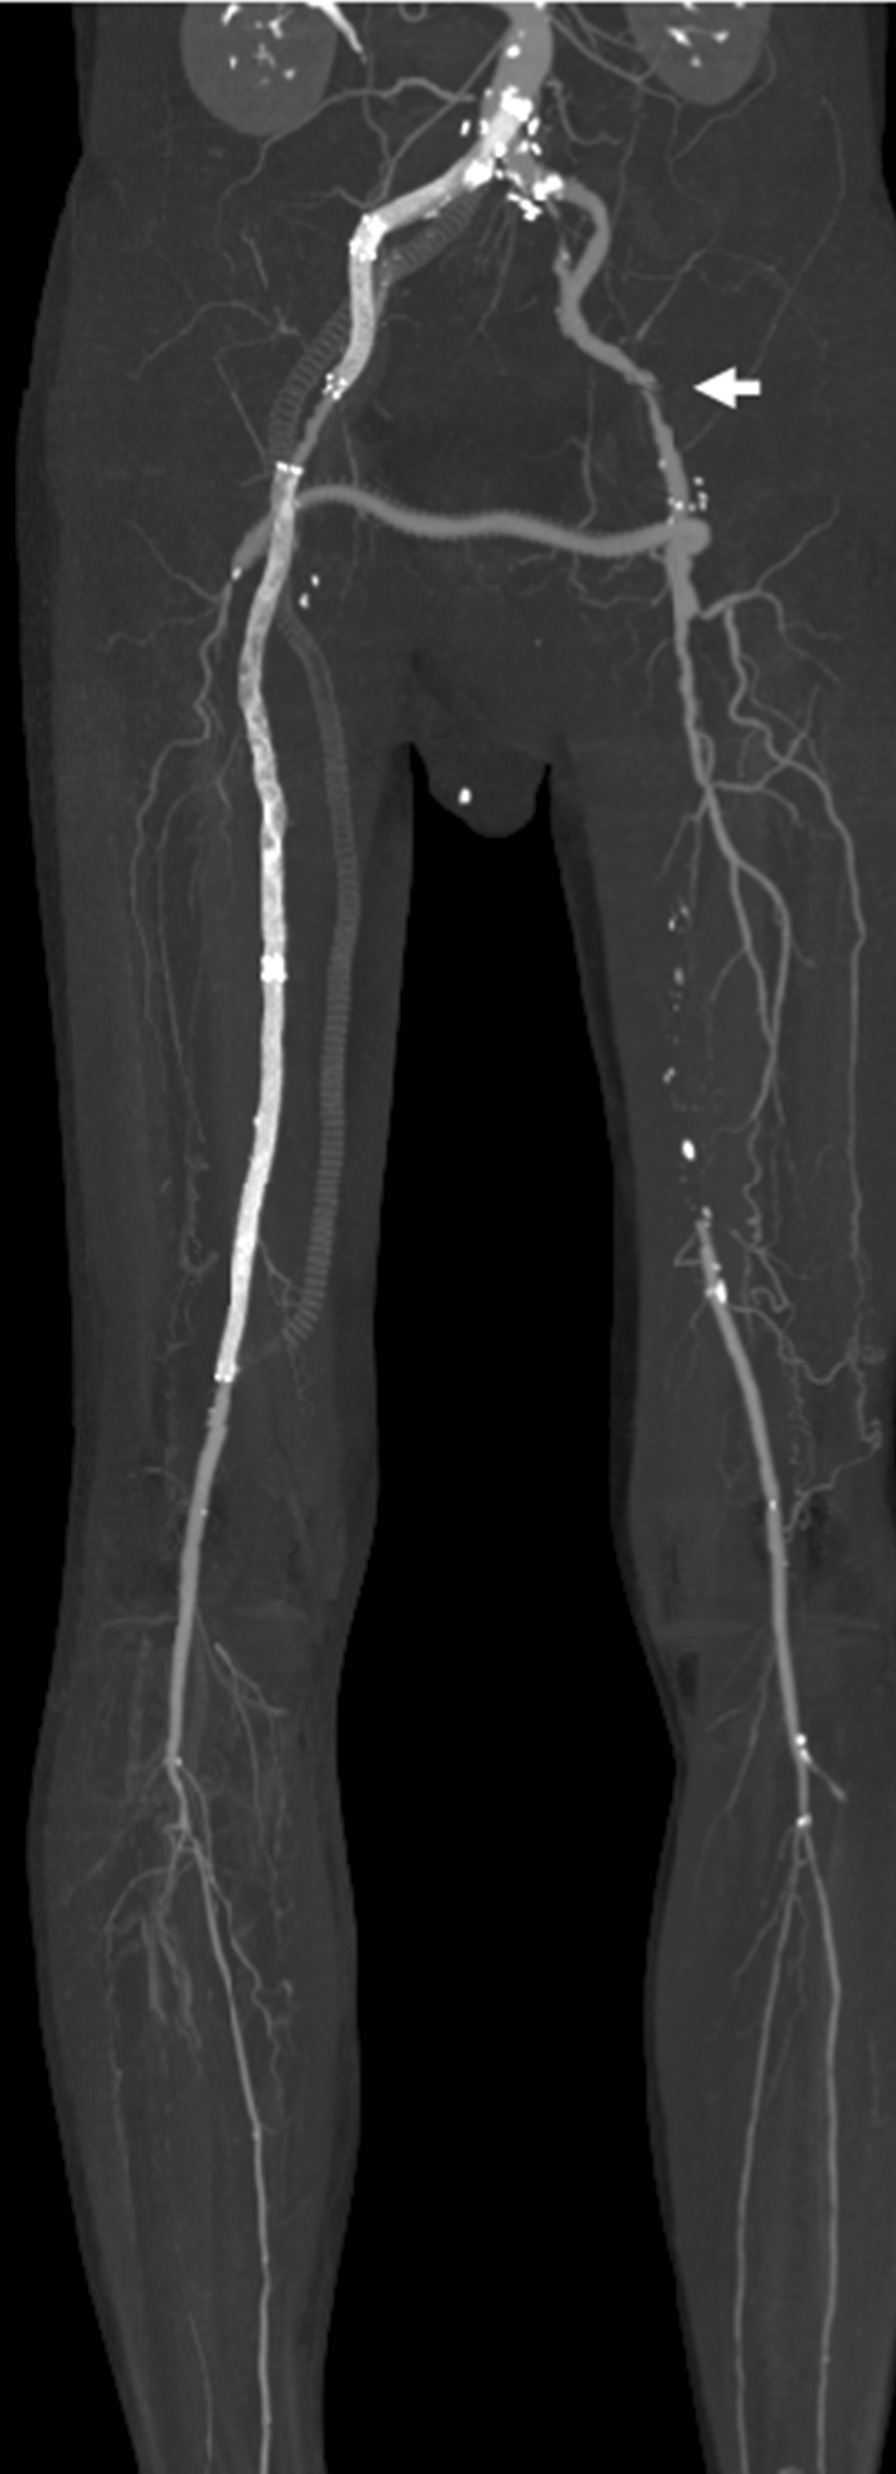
Multislice computed tomography (MSCT) after a rescue left common femoral-right deep femoral artery bypass. Good blood flow but remaining thrombus in the implanted stents and patency of femorofemoral bypass were found. The left external iliac artery had moderate stenosis (white arrow)
